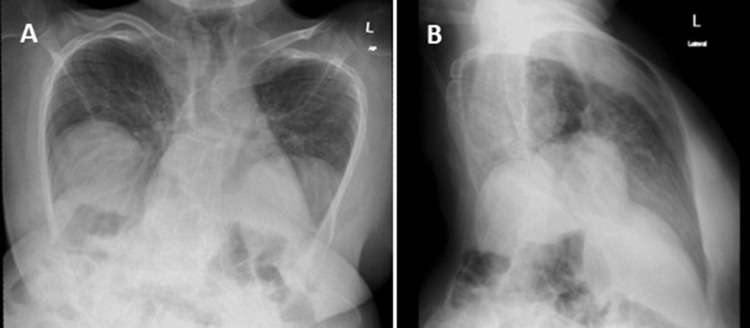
X-ray findings (A) Frontal anteroposterior, and (B) left lateral chest radiographs demonstrate fan-like rib orientation and abnormal thoracic contour, findings consistent with severe congenital spinal dysplasia, including pronounced thoracic lordosis and scoliosis
